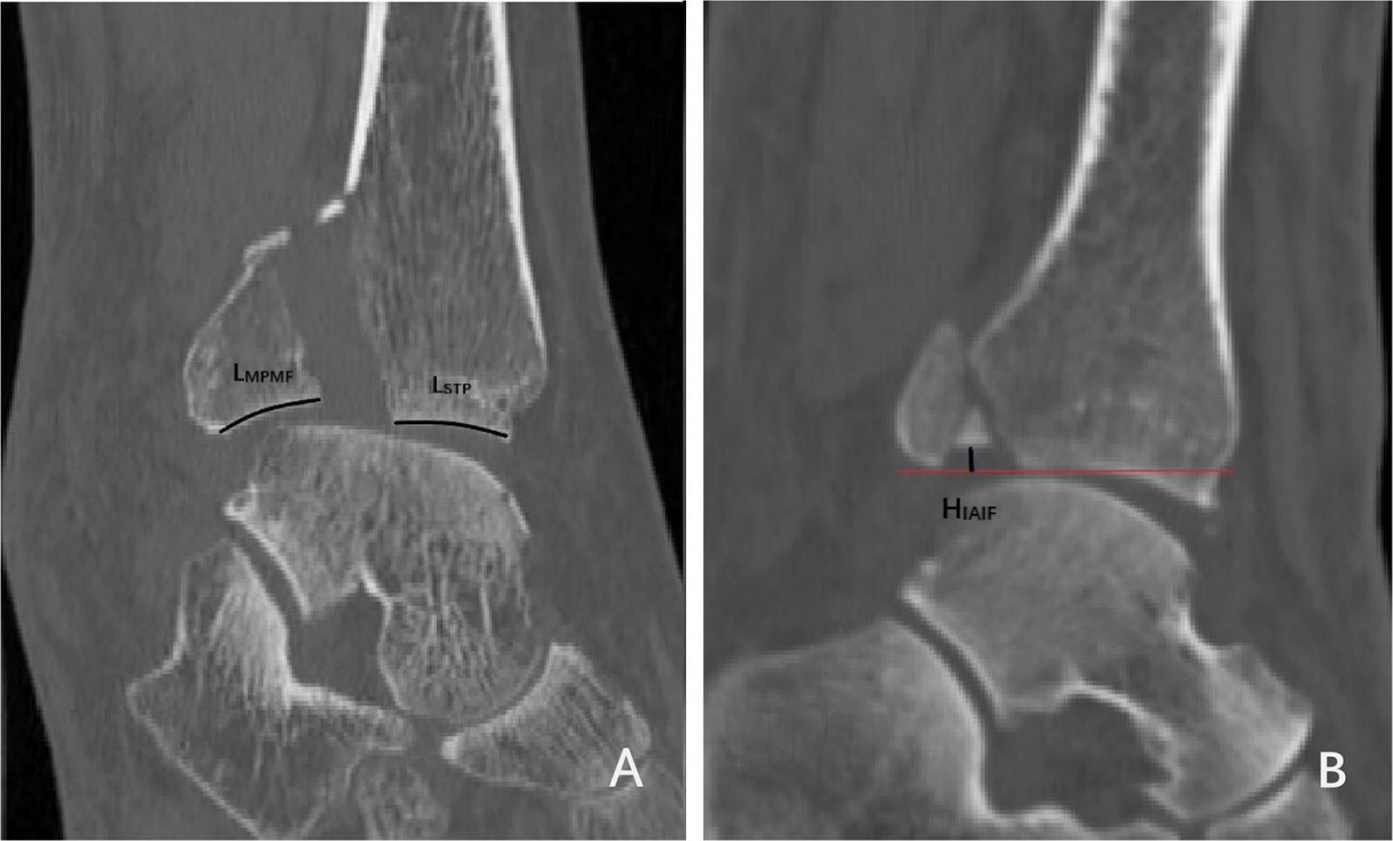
Example sagittal CT linear measurements. A Measured as the length of major posterior malleolar fragment (*L*_MPMF_, length of major posterior malleolar fragment) and stable tibial plafond (*L*_STP_, length of stable tibial plafond). B Measured as the displaced height of IAIF (*H*_IAIF_, height of IAIF) and the red line is the horizontal line of articular surface
